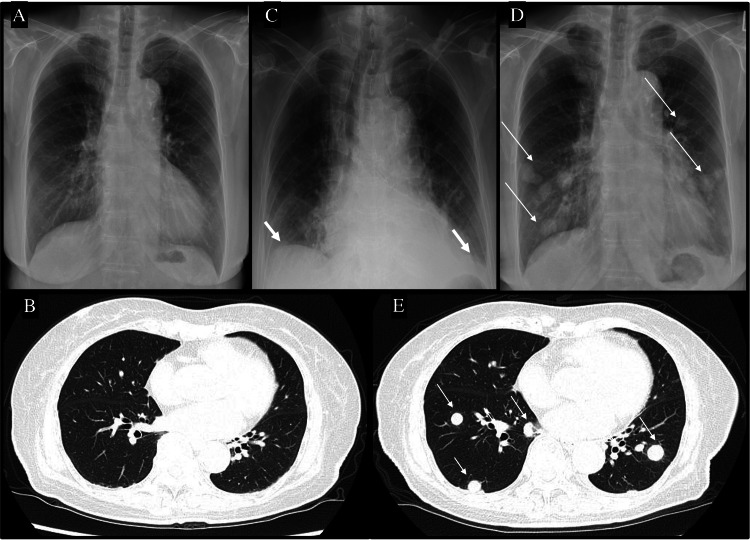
Rapid development of pulmonary metastases following surgery in an elderly uterine sarcoma patient A)    The preoperative chest X-ray showed no abnormal findings.
